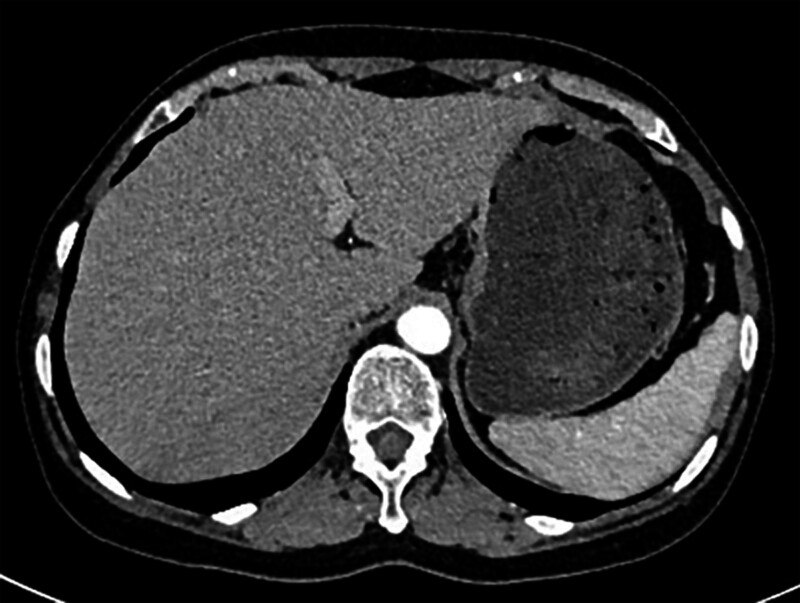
Two-year post-discharge follow-up computed tomography images show normal splenic size and morphology.
